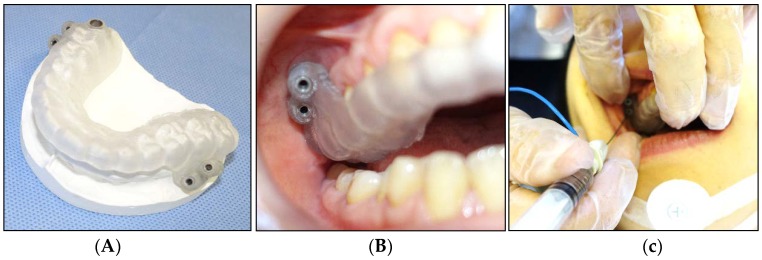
The needle guide using computer-aided design/computer-assisted manufacture-based data. Two points at the center of the inferior head of the lateral pterygoid muscle are selected, after checking the orientation and volume of the lateral pterygoid muscles on computed tomography [26]. A needle guide is fabricated using the stereolithographic method (A). The guide is inserted into the oral cavity and stabilized with the help of the teeth (B). A disposable hypodermic needle electrode is inserted through the metal sleeves (C).
